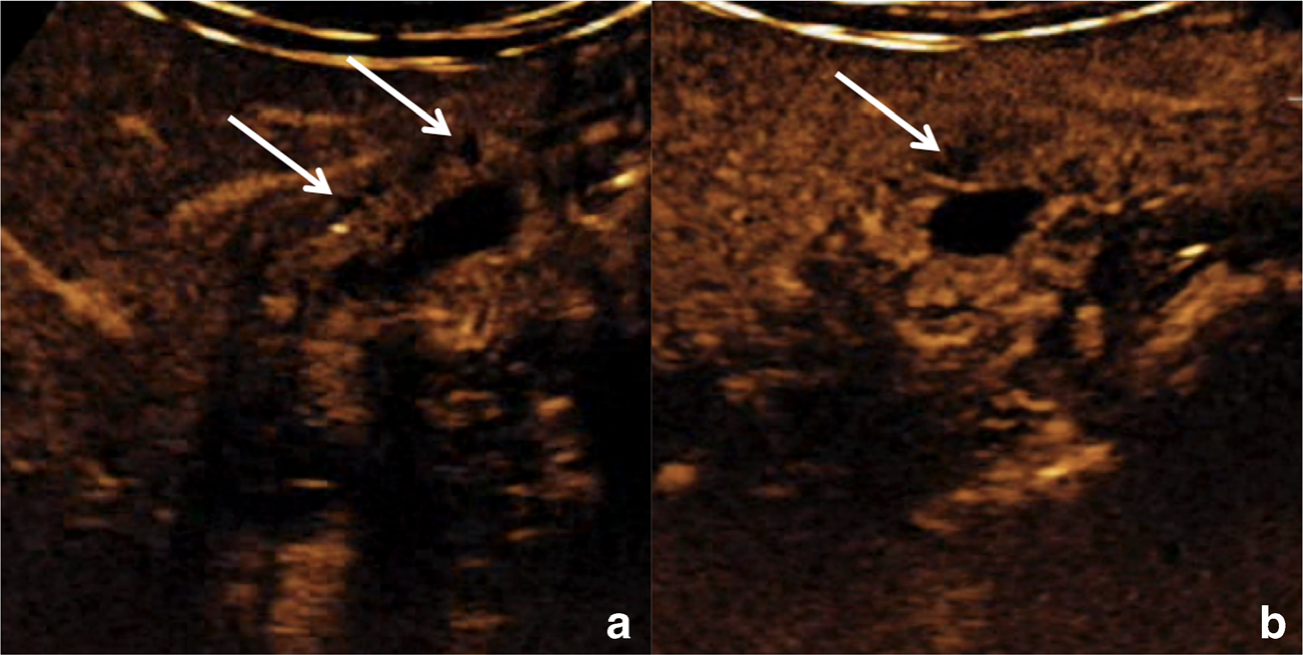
Gallbladder adenomyomatosis: typical contrast-enhanced ultrasound (CEUS) findings. On CEUS, the thickened gallbladder wall shows discrete contrast enhancement, whereas Rokitansky–Aschoff sinuses (*arrows*) appear as avascular structures during every phase of the exam
